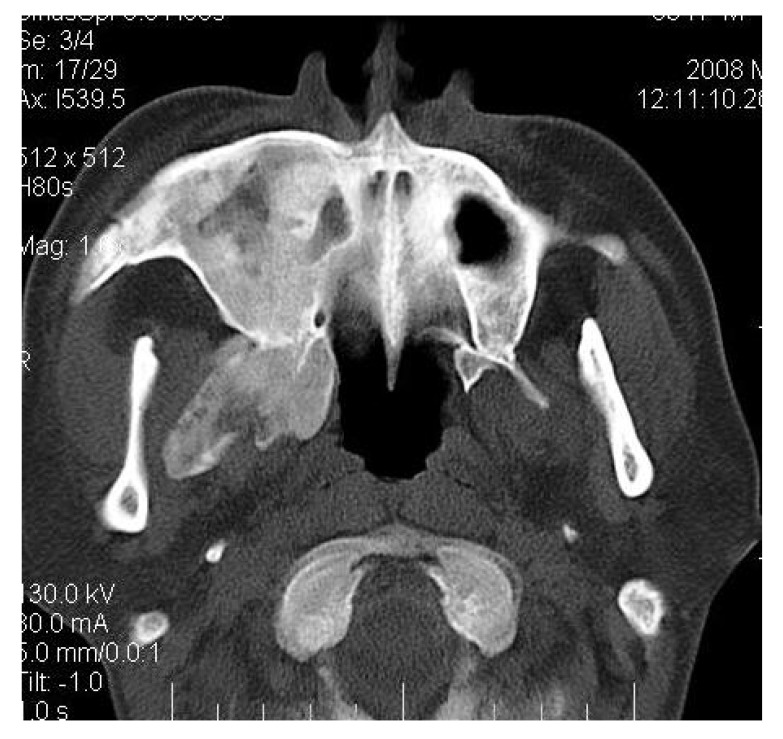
The Axial CT section showing the involvement of right side of the maxilla including the maxillary sinus, right zygoma, zygomatic arch, pterygoid plates.
